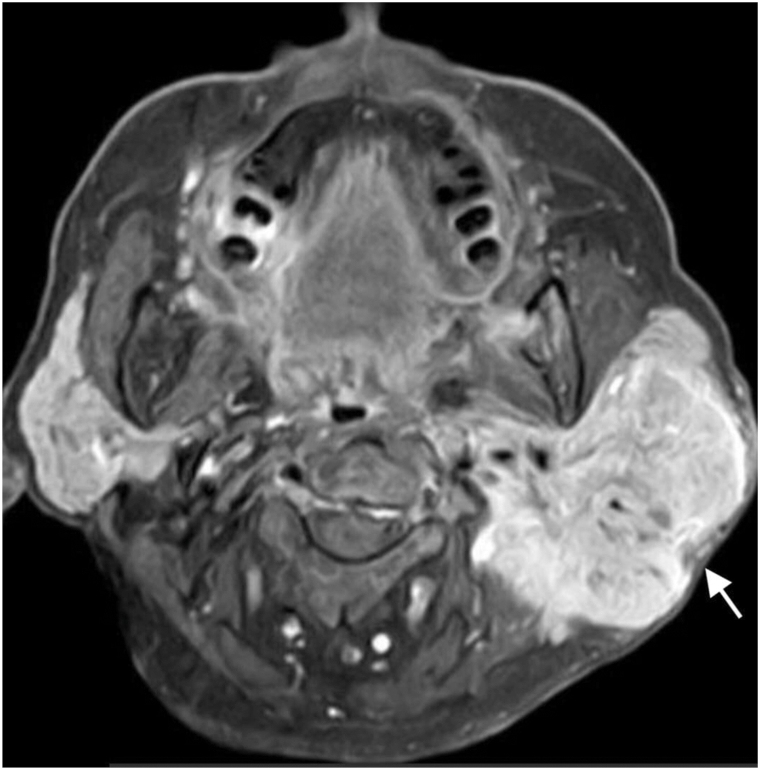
Contrast-enhanced axial magnetic resonance imaging neck at parotid level showing diffusely enlarged left parotid gland involving both lobes with T2 low signal, heterogeneous enhancement, cystic areas, and perineural spread (shown by *white arrow*). The lesion abuts the medial pterygoid muscle and mandible with medial parapharyngeal space compression, along with left cervical lymphadenopathy indicating locoregional malignant extension.
